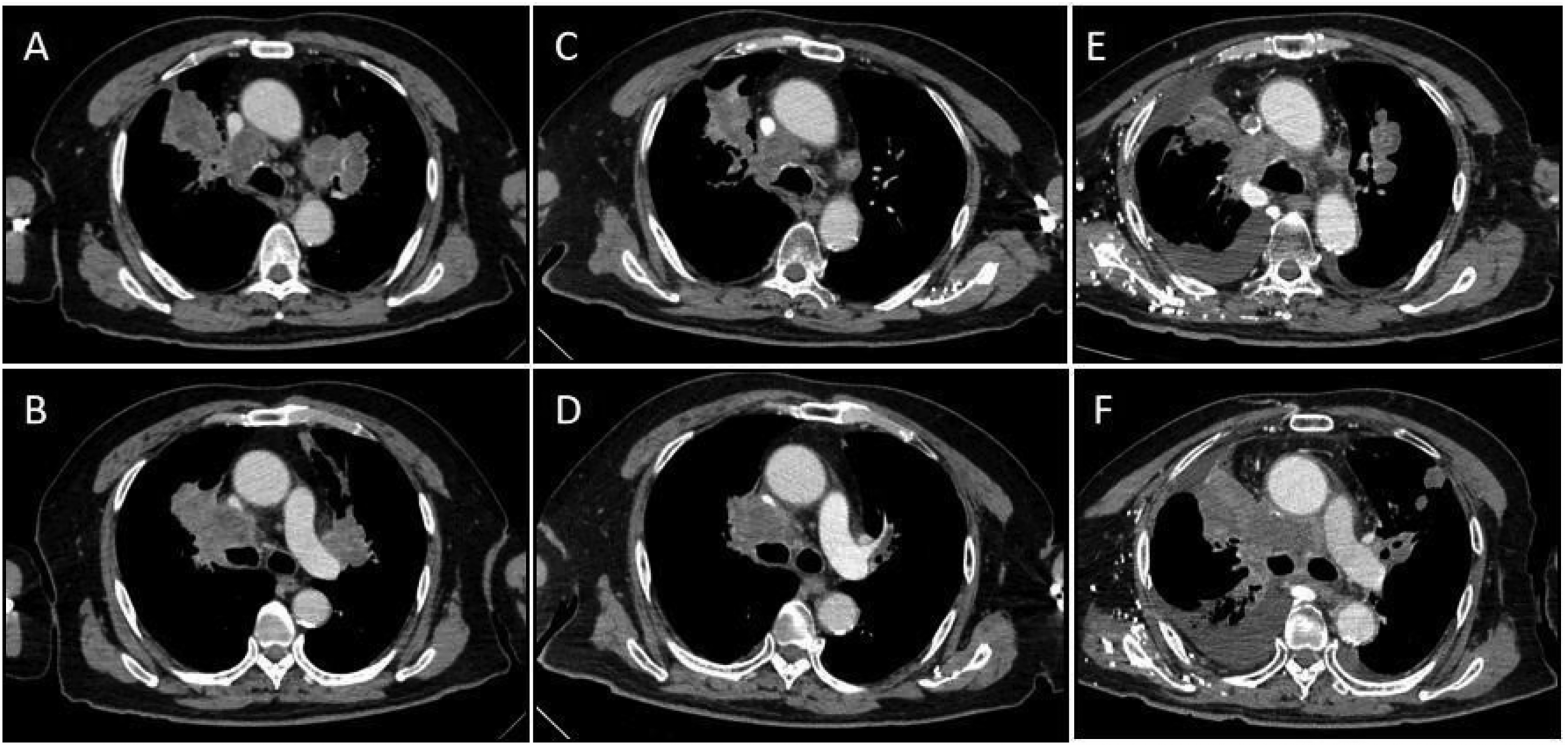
Comparison of chest CT images at different stages. (A,B) Initial diagnosis, (C,D) post-radiotherapy, (E,F) pre-immunotherapy with sintilimab.
